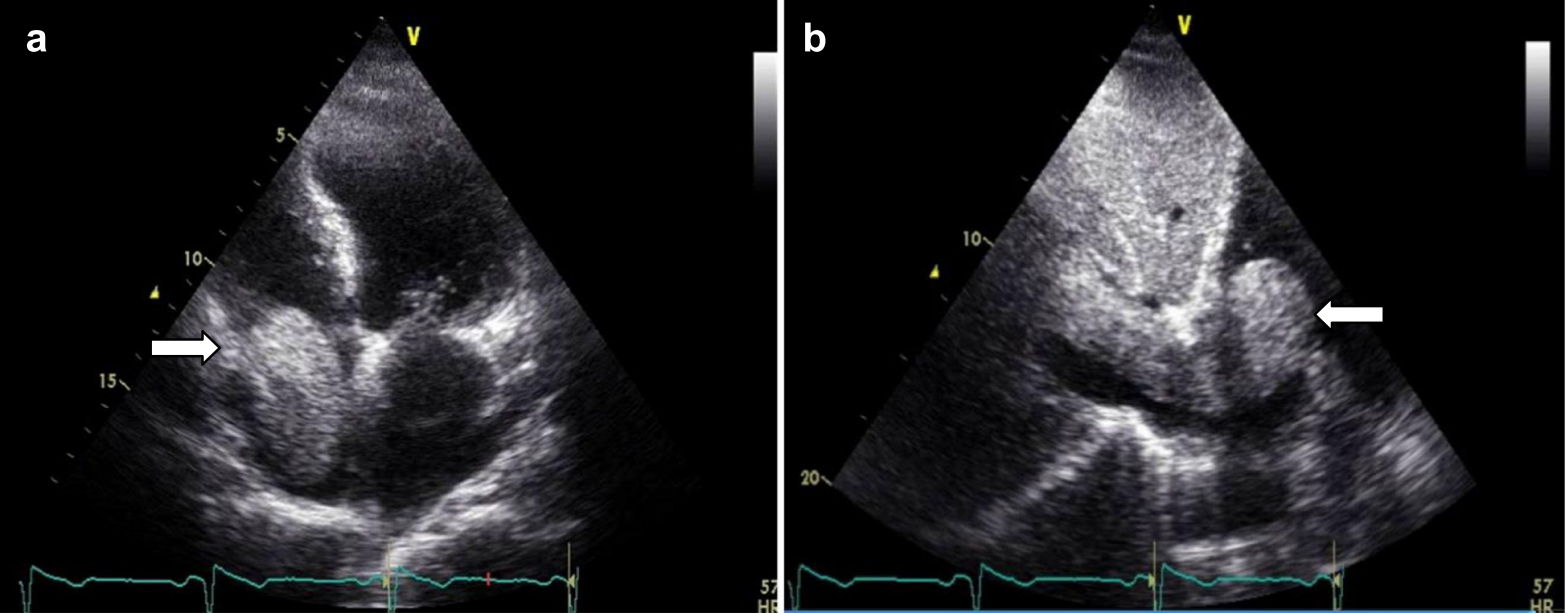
Apical four-chamber (a) and subcostal (b) views by transthoracic echocardiography showing the large mobile mass (*white arrow*) from inferior vena cava moving into right atrium and right ventricle
